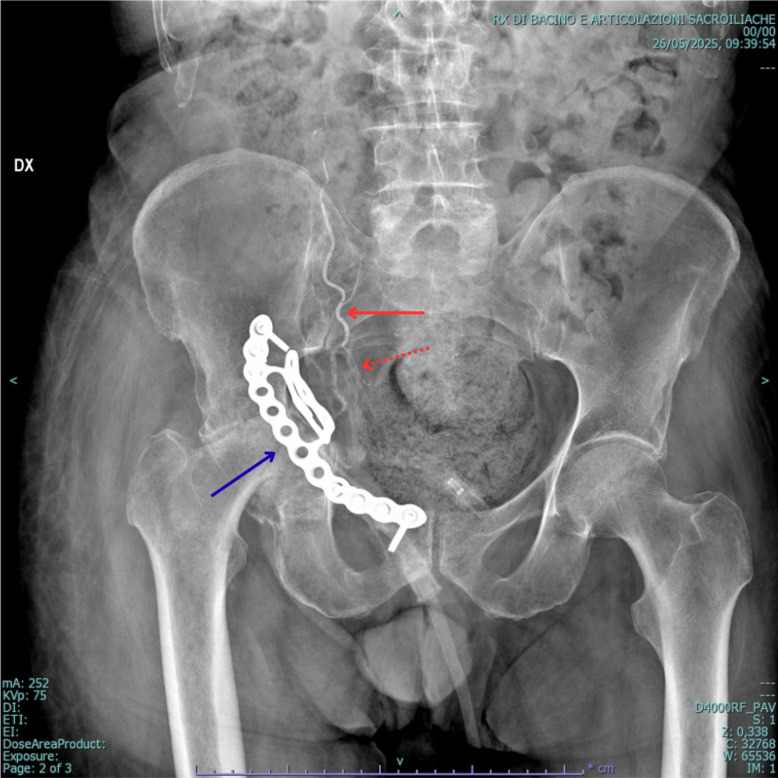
A 24-day X-ray examination of the pelvis showing osteosynthesis devices (continuous blue arrow) and radiopaque embolic material visible within the CM (dashed red arrow) and the inferior epigastric artery (continuous red arrow)
